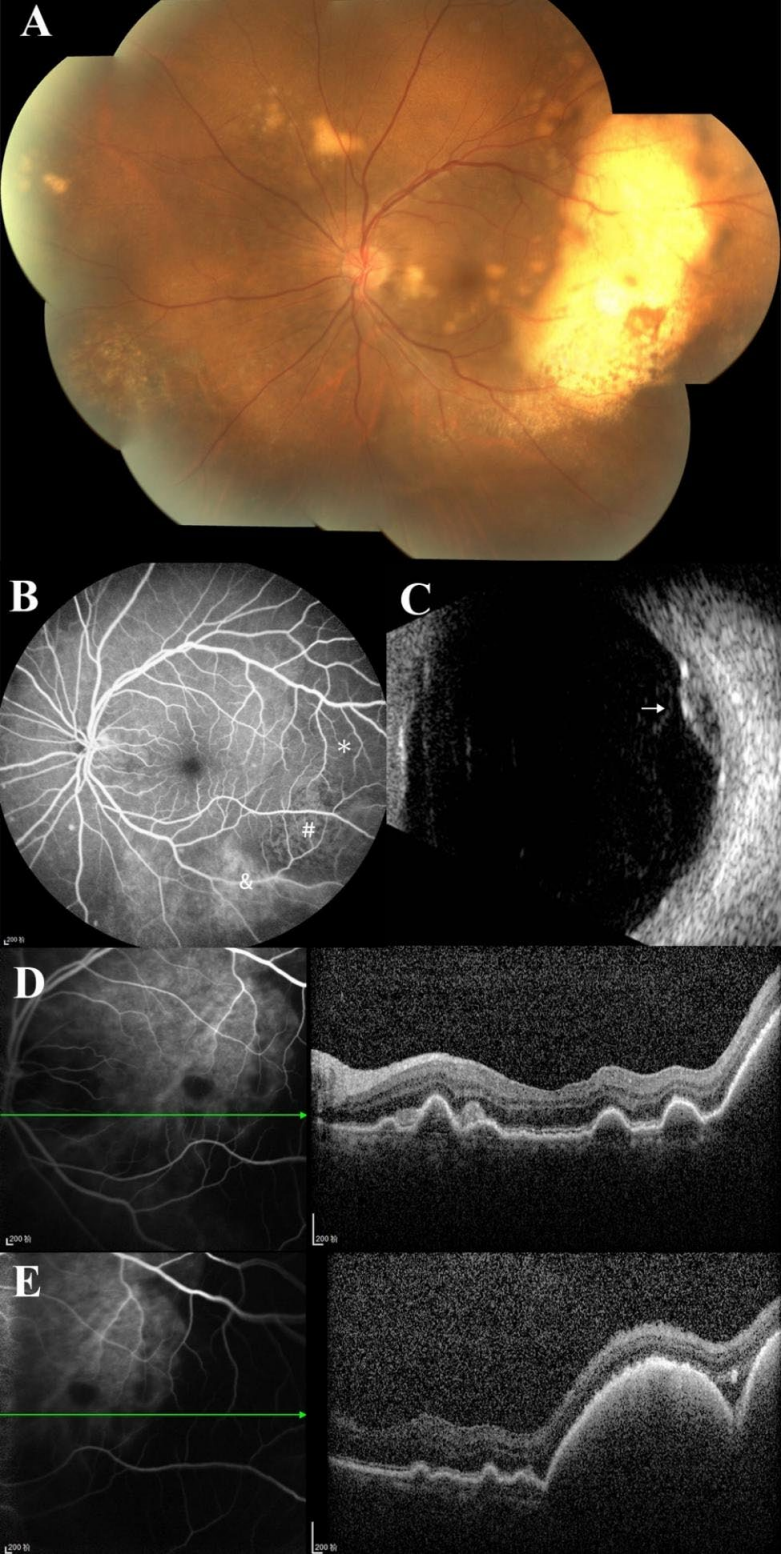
(A)Fundus examination showed an elevated yellowish sub-retinal lesion temporal to the macula, and scattered multifocal creamy lesions deep to retina; (B)FFA taken at 2 min showed hypo-fluorescence(*), mottled hyper-fluorescence(#) and hyper-fluorescence(&) area in the temporal mass(2-7o’clock); the leakage of the inferior part of the lesion(&) increased as the examination progressed; diffuse hyper-fluorescent spots at superior-nasal/inferior-nasal quadrants increased in both size and intensity; (C) Ultrasound of the left eye showed a small elevated lesion(arrow); (D) (E) OCT of the left eye showed multiple hyper-reflective lesions in the outer retina and beneath RPE at paramacular region, and domed retina temporal to the macula, nodular hyper-reflective signals between the Bruch’s membrane and RPE corresponding to fundus examination
